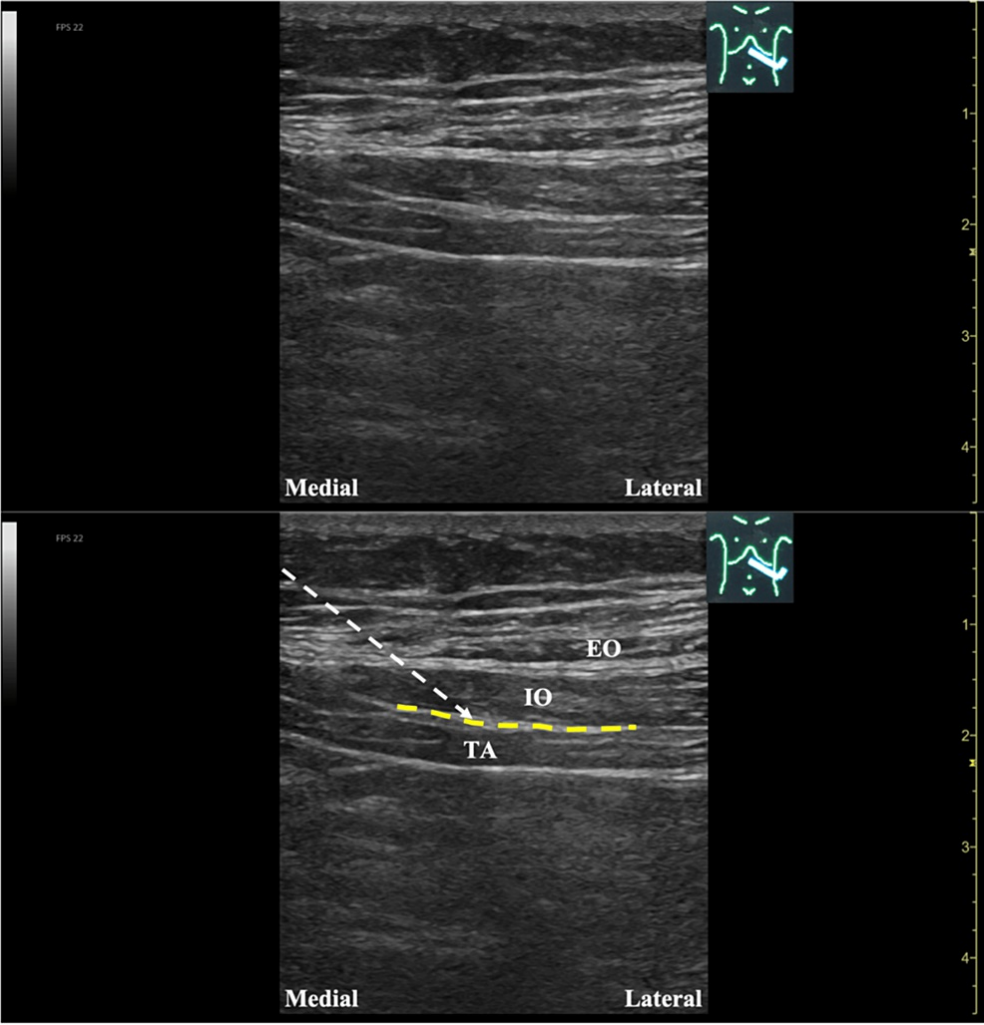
Ultrasound image at the time of puncture (the modality used was Venue GO R2 Final, GE Healthcare Japan Corporation, Tokyo, Japan). The white dashed line shows the needle trajectory. TA, transversus abdominis; IO, internal oblique muscle; EO, external oblique muscle; TAP, transversus abdominis plane (yellow dashed line)
